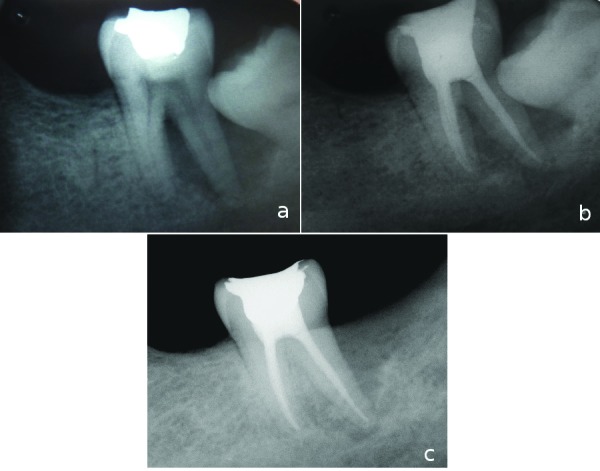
a) Initial radiograph of tooth #37. b) Final radiograph after obturation of the root canals. c) A year recall radiograph showing complete healing of the bone lesion.
